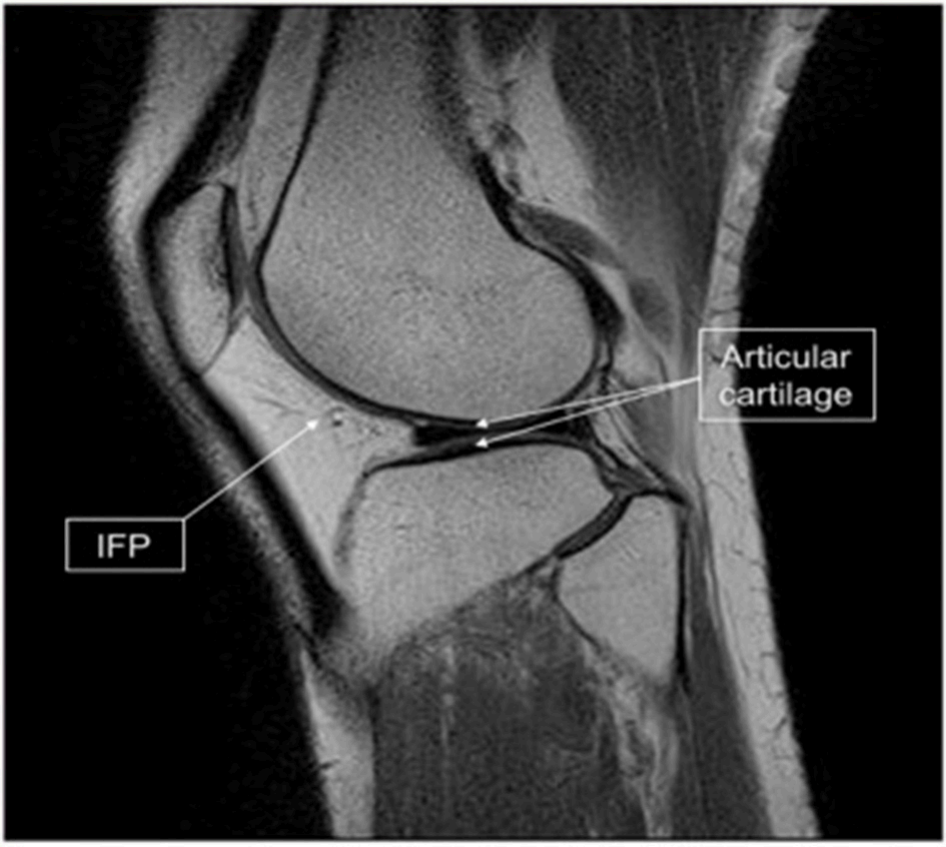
Infrapatellar fat pad (IFP) location and harvested tissue. Sagittal magnetic resonance imaging scan of the knee showing the relationship of the IFP (arrow) to the articular cartilage (double arrow). [Modified from and used with permission from Hindle et al. (22) under CC BL].
